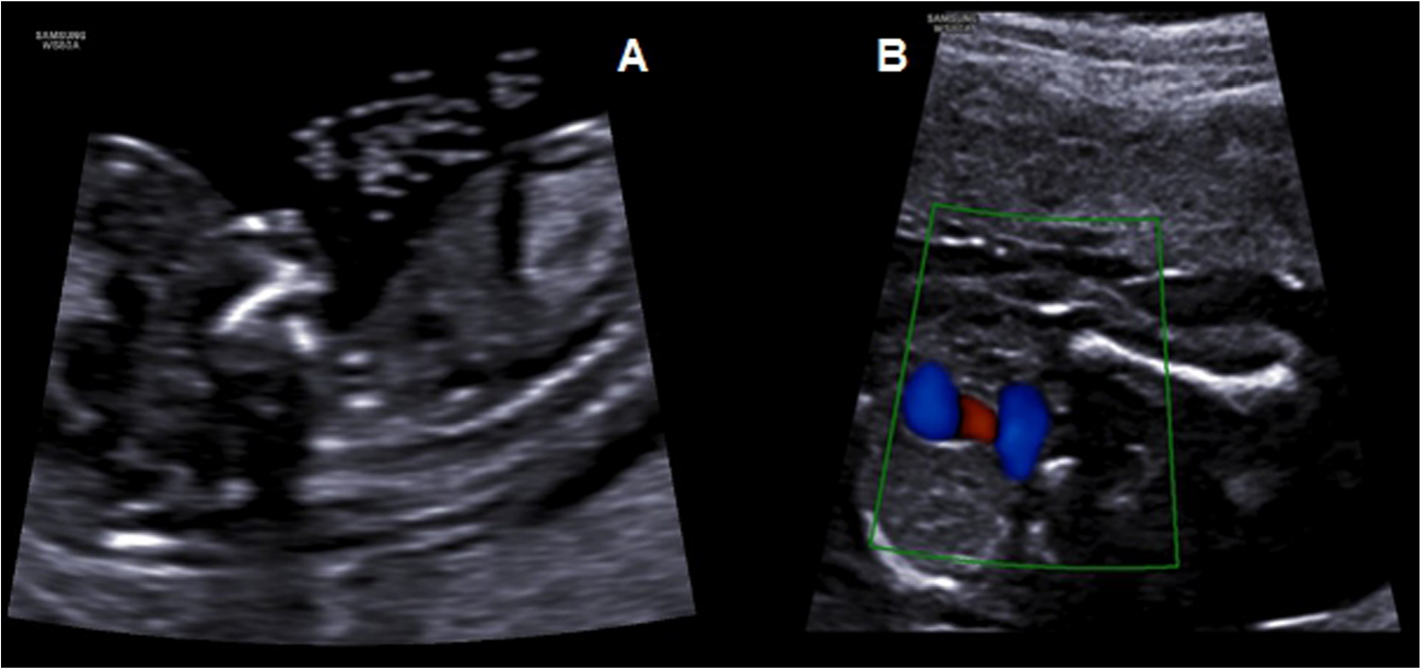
Case 2 ultrasound finding. a first-trimester ultrasound scan shows microretrognathia; b three vessels and trachea view shows the communication between aorta and pulmonary artery (aorto-pulmonary window)
